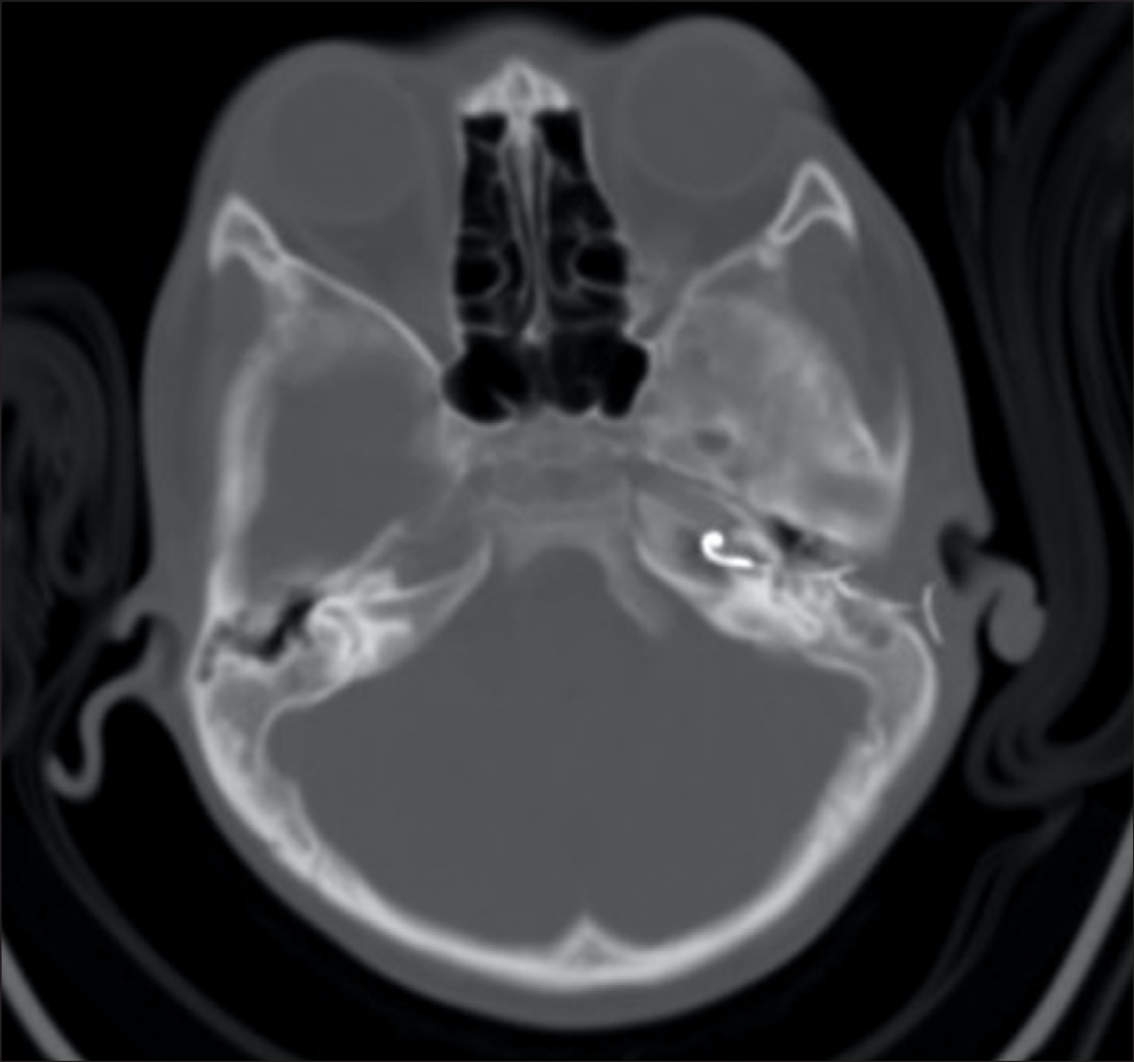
Axial CT image of a misplaced left cochlear implant electrode entering the carotid canal.
